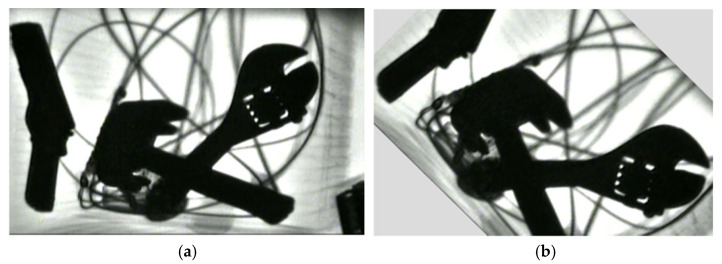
Affine transformation of prohibited items X-ray image. (a) The original captured X-ray image; (b) the affine transformation of (a).
